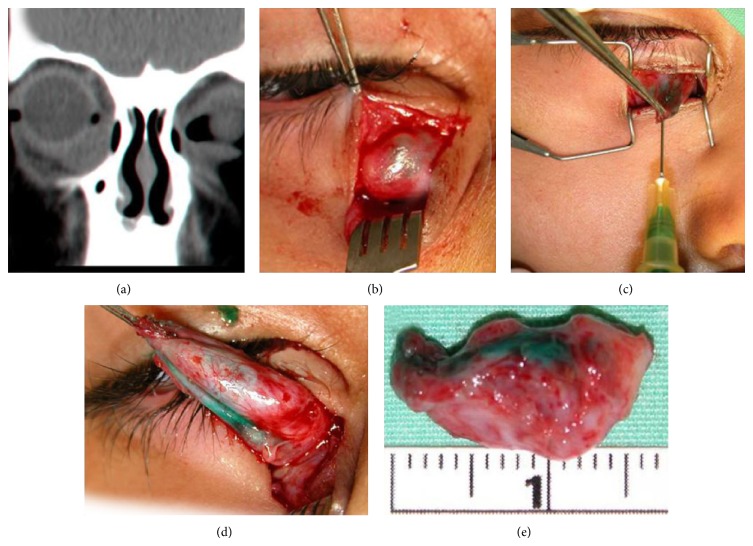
(a) Coronal CT scan showing a 2 cm homogenous inferomedial cystic mass in the right orbit. (b) Cyst exposure through a skin incision. (c) Intralesional injection of ICG. (d) Capsule demonstration and further exposure. (e) The completely excised cyst.
